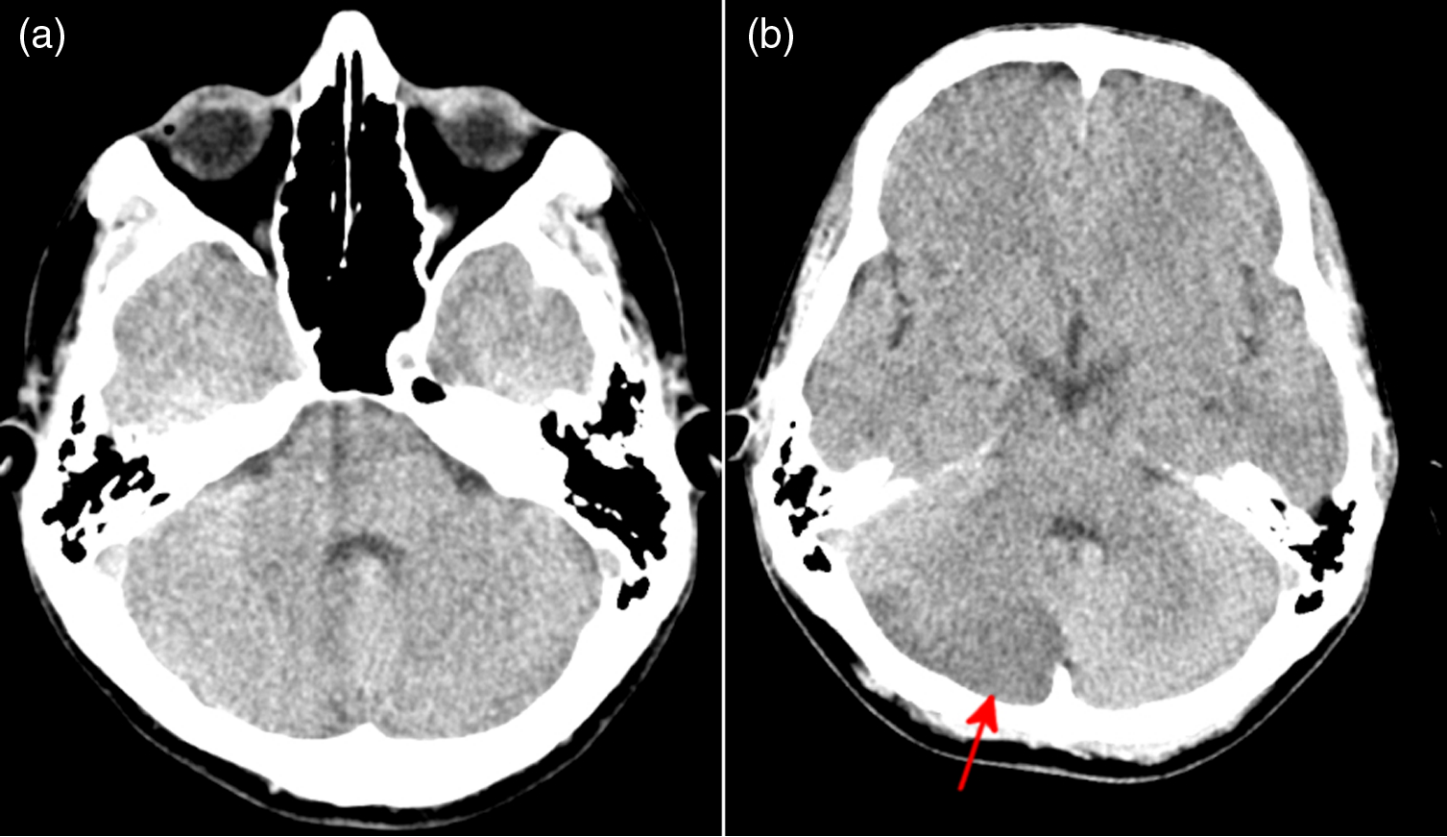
Computed tomography of the head on day of injury (a) just after initial injury showing no acute intracranial pathology, and (b) six hours after injury showing acute infarct involving the right cerebellar hemisphere (arrow).
